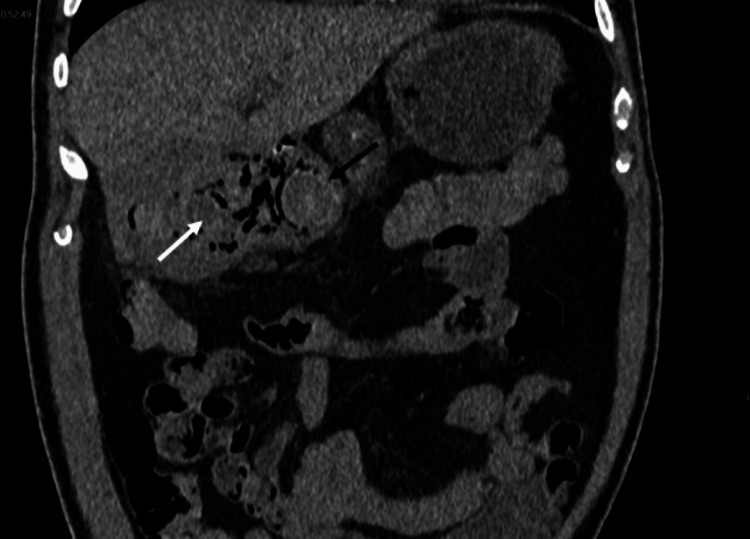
Coronal CT non-contrast, as previous image, demonstrates gallstone (black arrow) fistulated into duodenum with gas in the gall bladder (white arrow).
